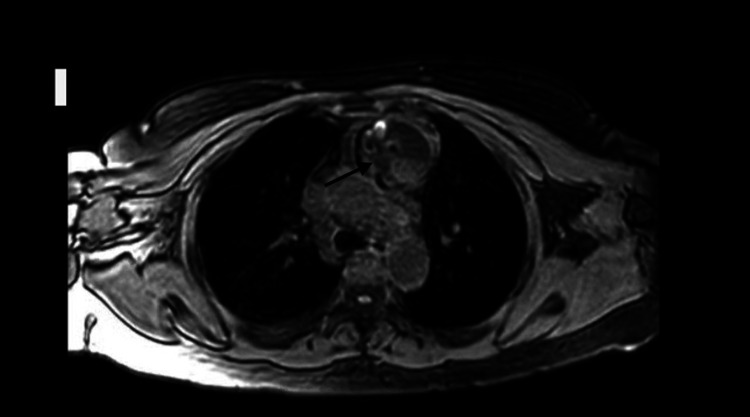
MRI of the chest with and without contrast confirming the presence of an anterior mediastinal mass measuring 5.9 x 7.4 x 8.5 cm. MRI: Magnetic resonance imaging
